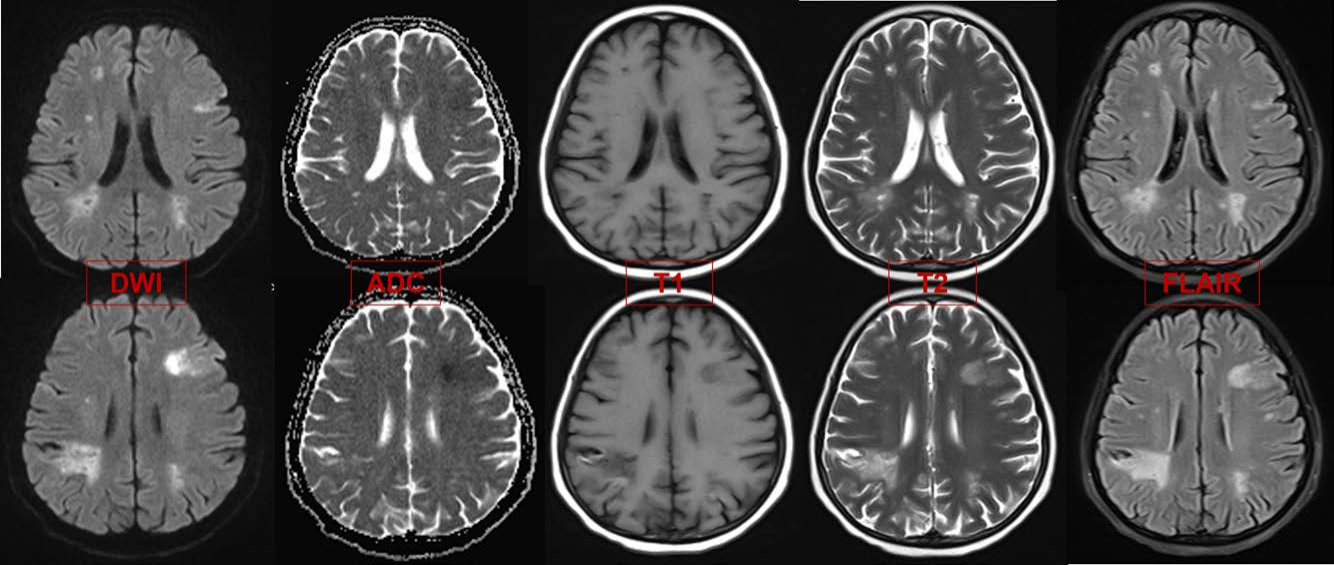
Cranial MRI showed new lesions in bilateral frontal lobe and left medial temporal lobe. MRI = magnetic resonance imaging.
